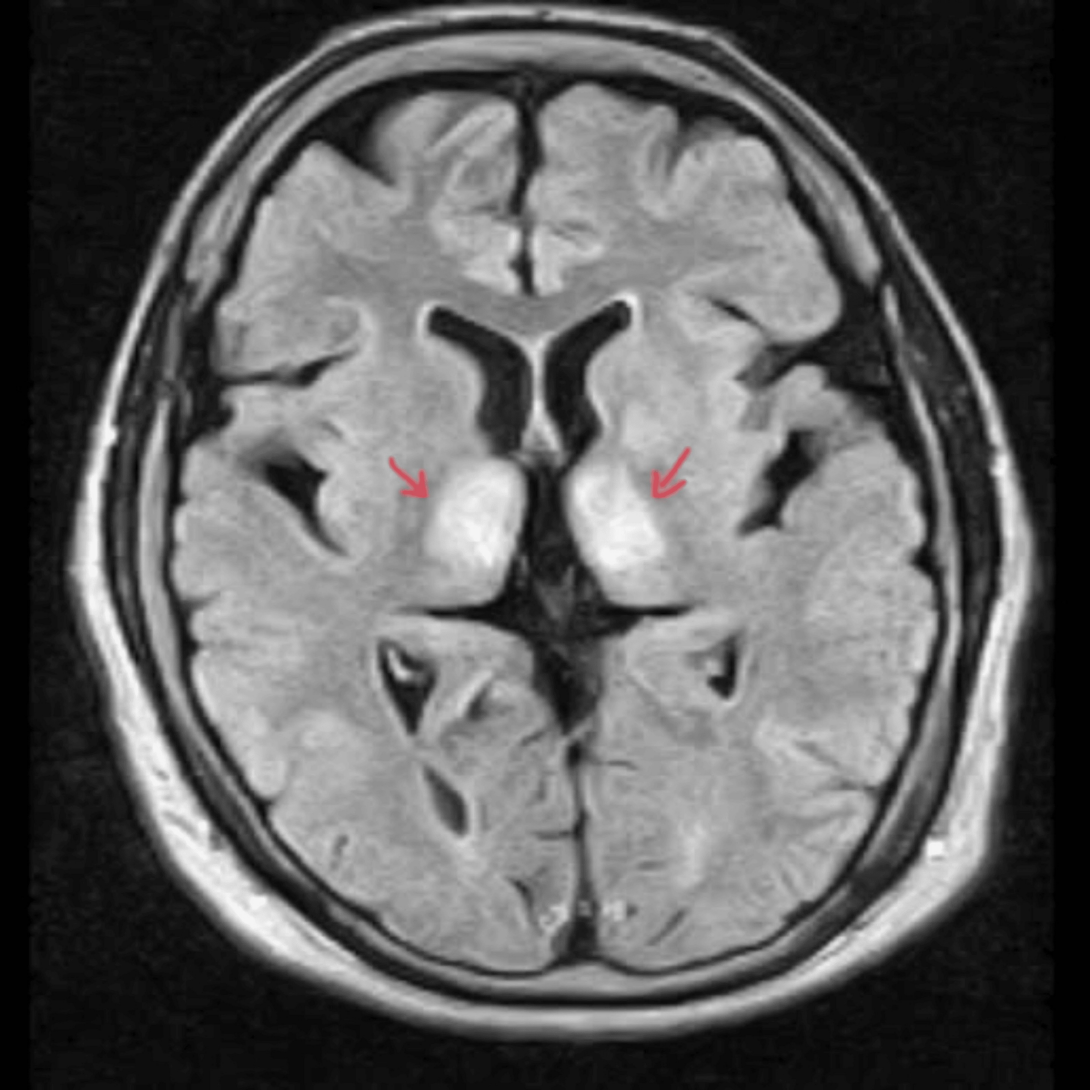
MRI of the brain showing DWI hyperintensities involving the dorsal medial and pulvinar nuclei of thalami and perimesencephalic regions DWI, diffusion-weighted image
